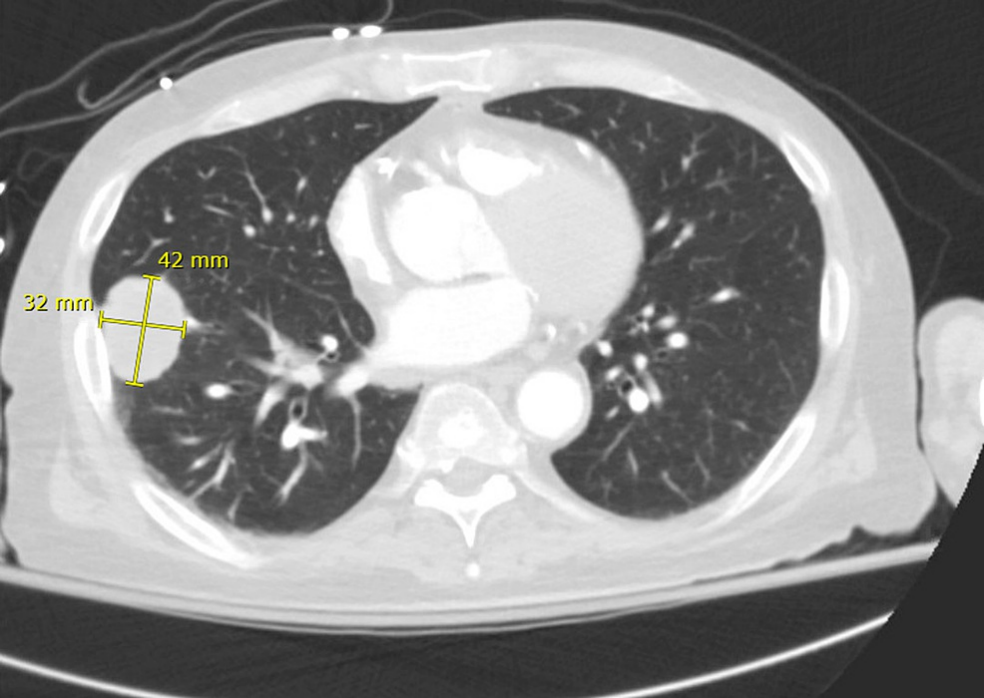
Axial view of CT chest demonstrating a right lower lobe mass in the basal lateral segment measuring 3.2 x 4.2 cm.
